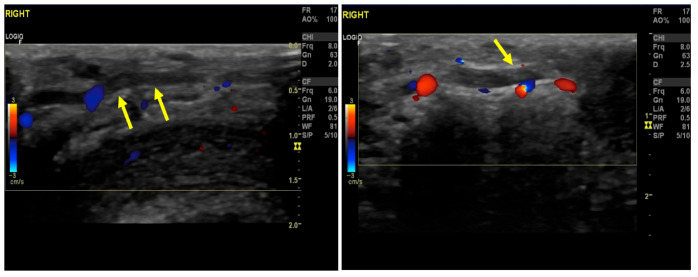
Longitudinal Color-Doppler ultrasound of the penis showing endoluminal echogenicity consisting of the thrombus (arrows), along with the absence of vascular flow signals within the dorsal vein of the penis. The examination was performed with the patient in a supine position, using a vascular linear probe (General Electric, Boston, MA, USA) (frequency range: 12–4 MHz). These images are pathognomonic and, along with the history and clinical examination, are sufficient to diagnose Mondor disease [1,2]. Despite its distinctive clinical and imaging features, Mondor disease remains under-recognized, constituting a definitive treatment algorithm impossible to be designed. The current literature supports conservative treatment, which includes NSAIDs and sexual abstinence, and reserves anticoagulation treatment for cases with proven thrombophilia or recurrent disease. Surgical excision should be considered only in refractory cases [3]. As our patient had no history of thrombophilia, sexual abstinence and pain relief management with NSAIDs were offered. After 6 weeks, the penile induration had been resolved.
